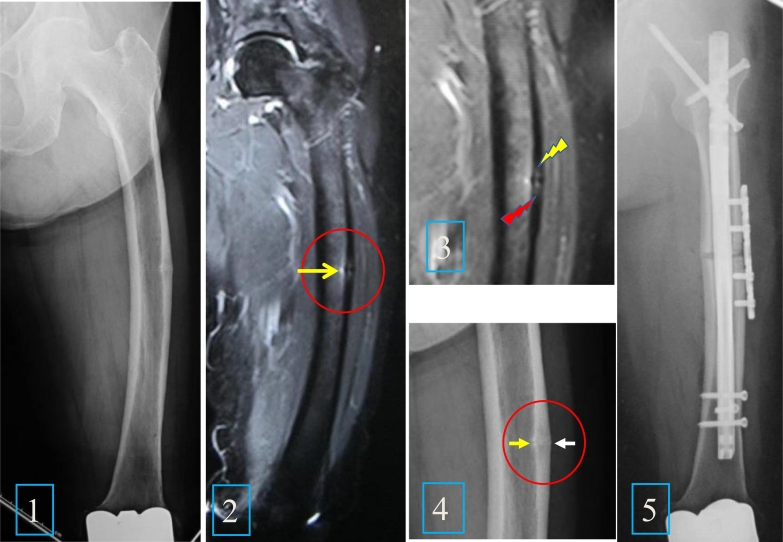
Preoperative X-ray, MRI and post-operative X-ray. 1-1. Preoperative X-ray of the left femur, with lateral bowing and hypertrophy of the lateral cortex of both periosteal and endocortical surfaces of the midshaft. 1-2. Preoperative MRI of the left femur, showing high intensity area in the bone marrow adjacent to the mid-lateral cortex (yellow arrow). 1-3. Preoperative MRI of the left femur, showing high intensity area in the intracortical region of the mid-lateral cortex (upper end: yellow arrow; lower end: red arrow). 1-4. Preoperative X-ray of the left femur. An enlarged image of the midshaft, indicating the hypertrophy of both periosteal (white arrow) and endocortical (yellow arrow) surfaces. 1-5. Postoperative X-ray of the left femur: lateral bowing was corrected and fixed with intramedullary rodding and plate fixation. (For interpretation of the references to colour in this figure legend, the reader is referred to the web version of this article.)
